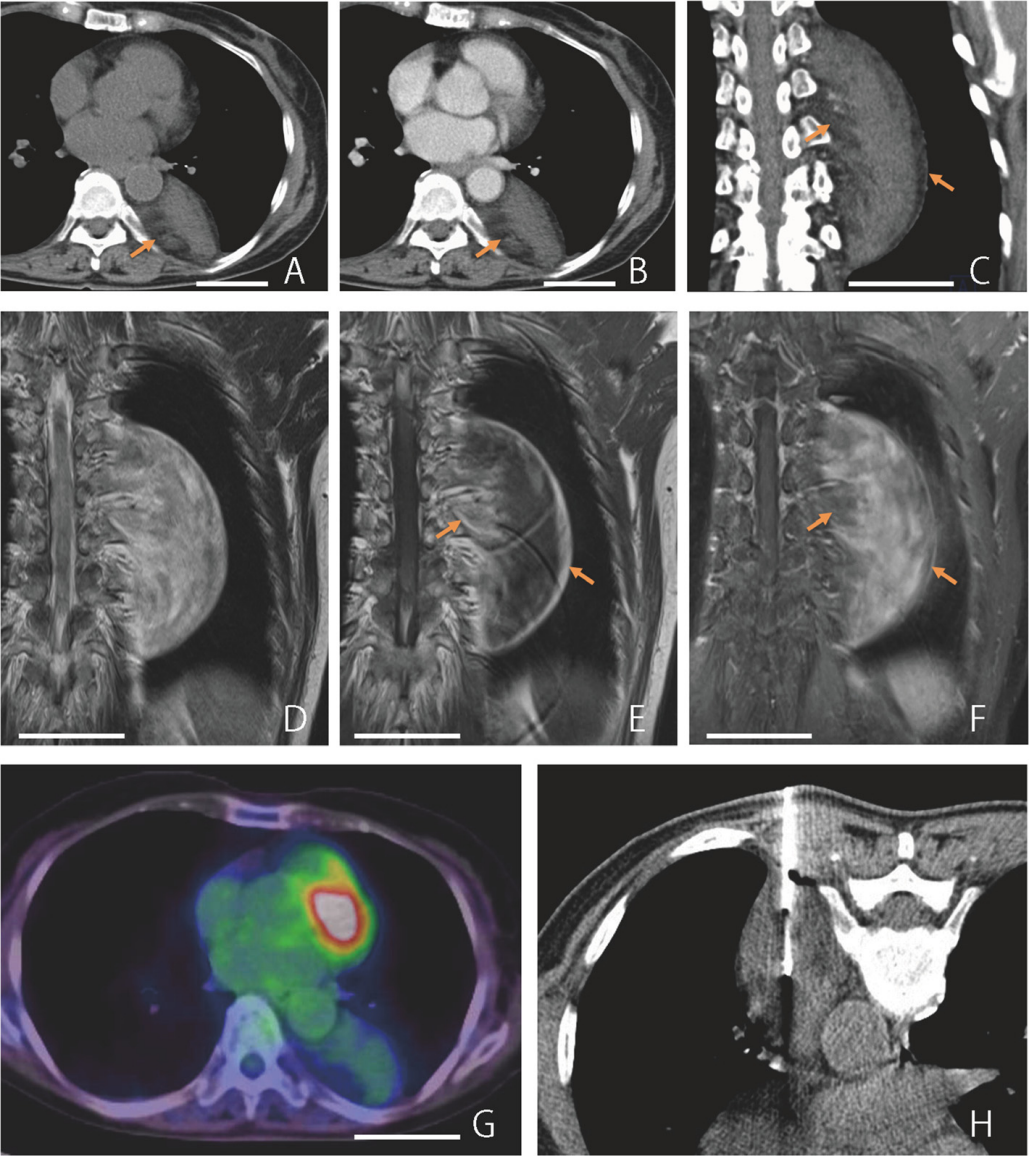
Radiographic images of the tumor. (A-C) Computed tomography images. A well-demarcated mass located in the left posterior mediastinum can be seen. The central portion of the tumor shows soft tissue density (27 Hounsfield unit, HU) on precontrasted computed tomography images (A). The late-phase contrast-enhanced computed tomography images (B, C) demonstrate slight or mild heterogeneous enhancement (35 HU). (D-F) Frontal parallel plane of magnetic resonance images. The tumor shows intermediate to high signal intensity on T2-weighted image (D), low signal intensity on T1-weighted image (E), and slight or mild heterogeneous enhancement on contrast-enhanced fat-suppressed T1-weighted image (F). The tumor appears to be in close contact with several intervertebral foramina. (G) 18F-2-fluoro-2-deoxyglucose-positron emission tomography/computed tomography scan at almost the same level as A and B. (H) Computed tomography-guided needle biopsy. The biopsy needle is inserted in the central portion of this mass. The orange arrows seen in A, B, C, E, and F indicate intratumoral fat tissue. The white bars seen in A to G represent 5cm.
